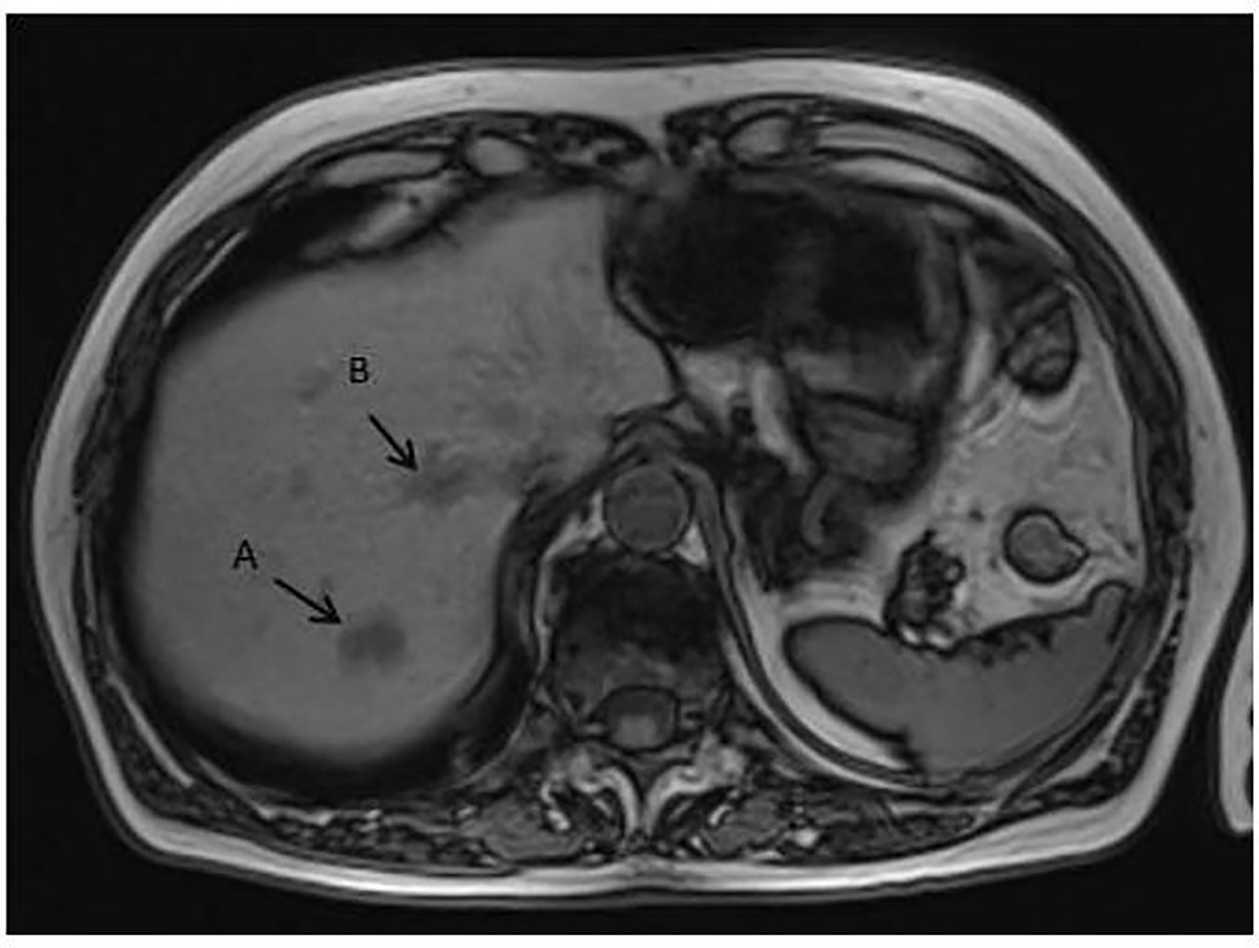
MRI liver revealed two metastases: (A) in segment VII and (B) straddling segments V and VI.
